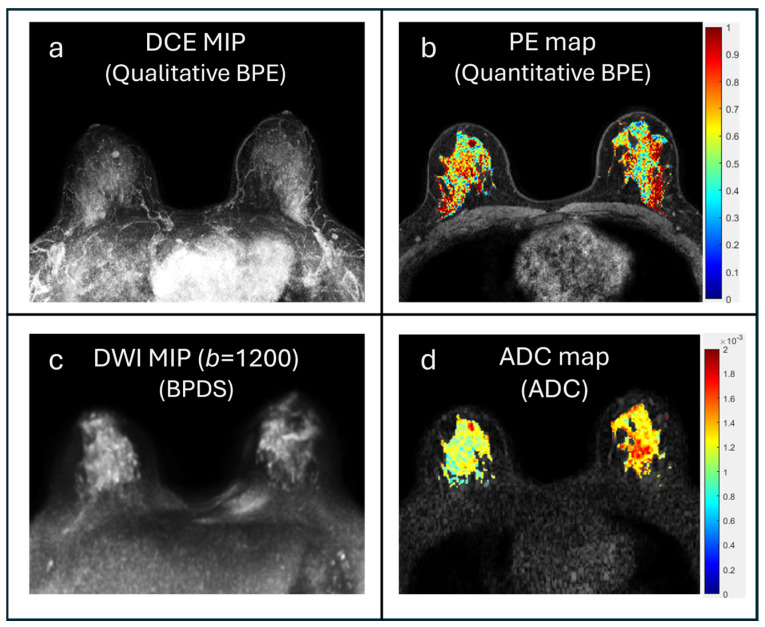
Imaging markers extracted from multiparametric MRI. Examples are shown from a 37-year-old woman with dense breasts, with qualitative BPE assessed as marked ((a), DCE MIP shown) by radiologists. Quantitative BPE measures calculated using whole-breast FGT segmentation and PE map (b), yielding a median BPE 47.7% and BPE volume of 262 cm^3^. Qualitative BPDS assessed as marked by a radiologist from b = 1200 s/mm^2^ DWI (c) and associated ADC map (d), with median ADC measured for the whole-breast FGT of 1.41 × 10^−3^ mm^2^/s.
